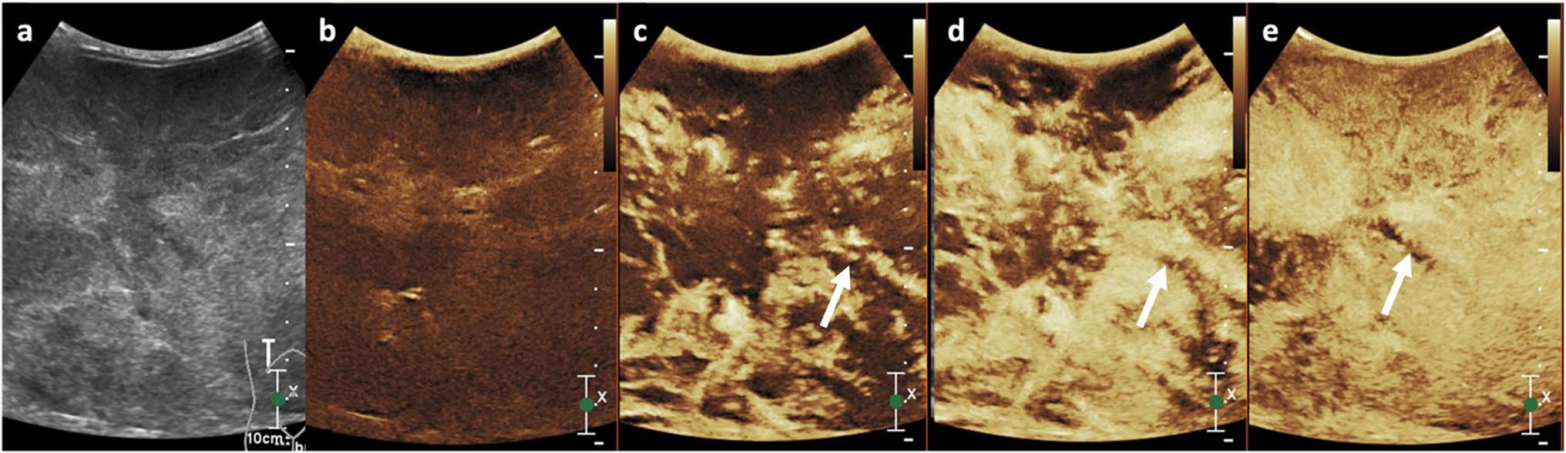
Follow-up contrast-enhanced ultrasound of the same 2-year-old girl, who developed acute kidney injury after chemotherapy treatment of the hepatic angiosarcoma. a Transverse greyscale (i.e. non-contrast-enhanced) ultrasound showed a large predominantly hypoechoic left lobe hepatic mass. b–e Transverse contrast-enhanced ultrasound in contrast-only mode in the arterial phase of the same mass at 5 s (b), 10 s (c), and 15 s (d), and in the portal venous phase 60 s after injection of the ultrasound contrast agent (e), shows the same progressive variegated multi-nodular enhancement seen on MR scan in Fig. 2. Similar hypoenhancing striations (white arrow) were observed, suggesting fibrous septation. Reprint with permission: [85]
